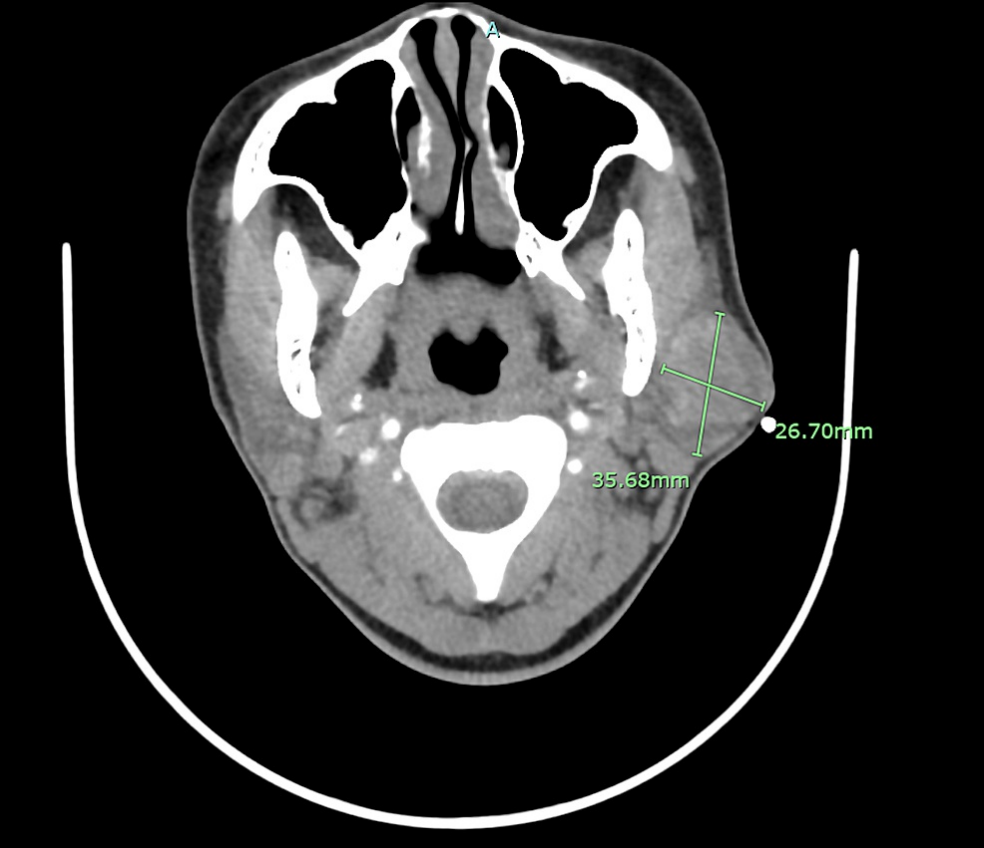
CT scan of soft tissue of neck with contrast demonstrating a solid nodule in the superficial portion left parotid gland measuring 3.56 x 2.67 cm that appeared to be homogeneous and had a well-defined margin.
